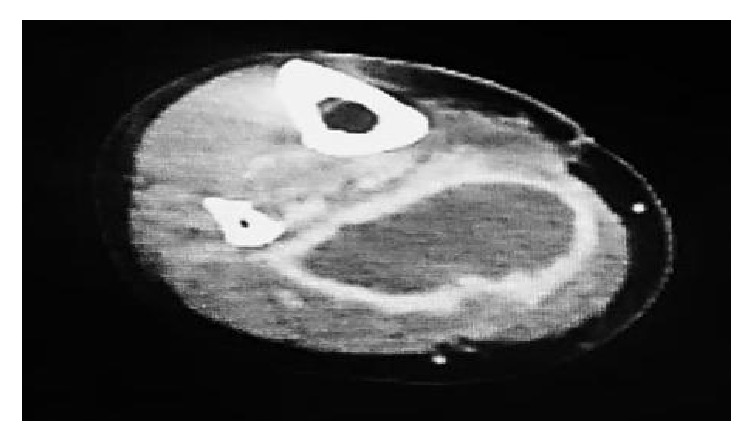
Contrast-enhanced CT on day 32.
